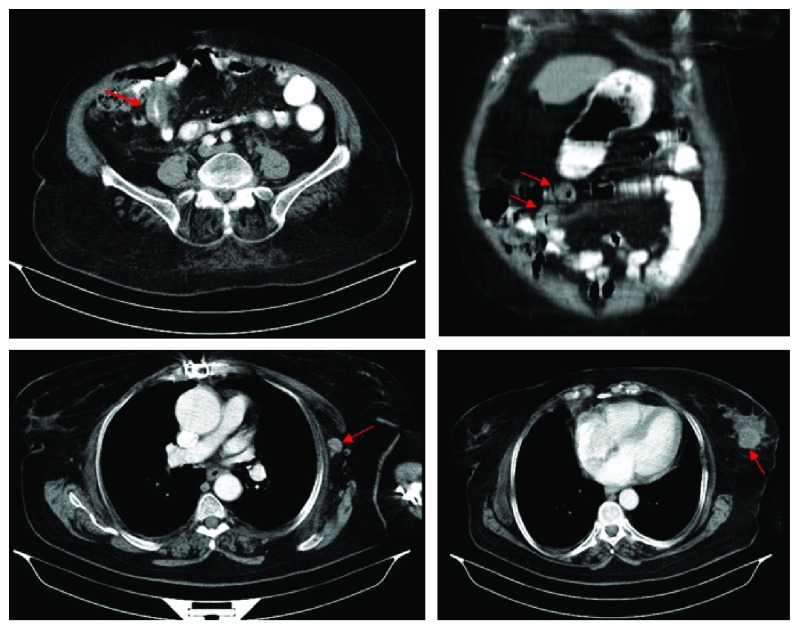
Contrast-enhanced abdominal and pelvic CT. (A) Circumferential thickening of an ileal loop with adjacent fat stranding in Sagittal (1) and Coronal (2) view. (B) LAP on left axilla with malignant feature measuring 18×16mm (3) and 32×26 mm mass in left breast (4).
